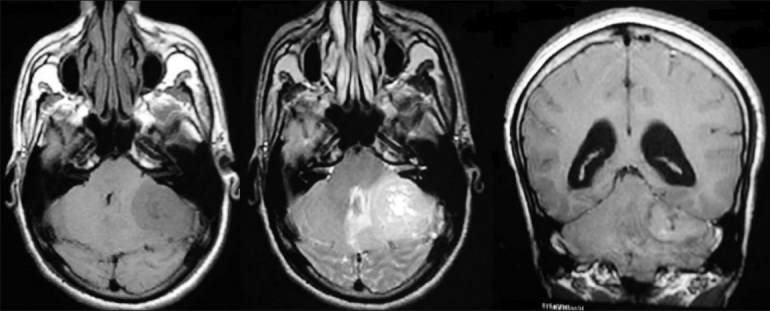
MRI of the brain axial T1, T2 and coronal sections showed a left CP angle lesion with broad-based tentorial attachment
